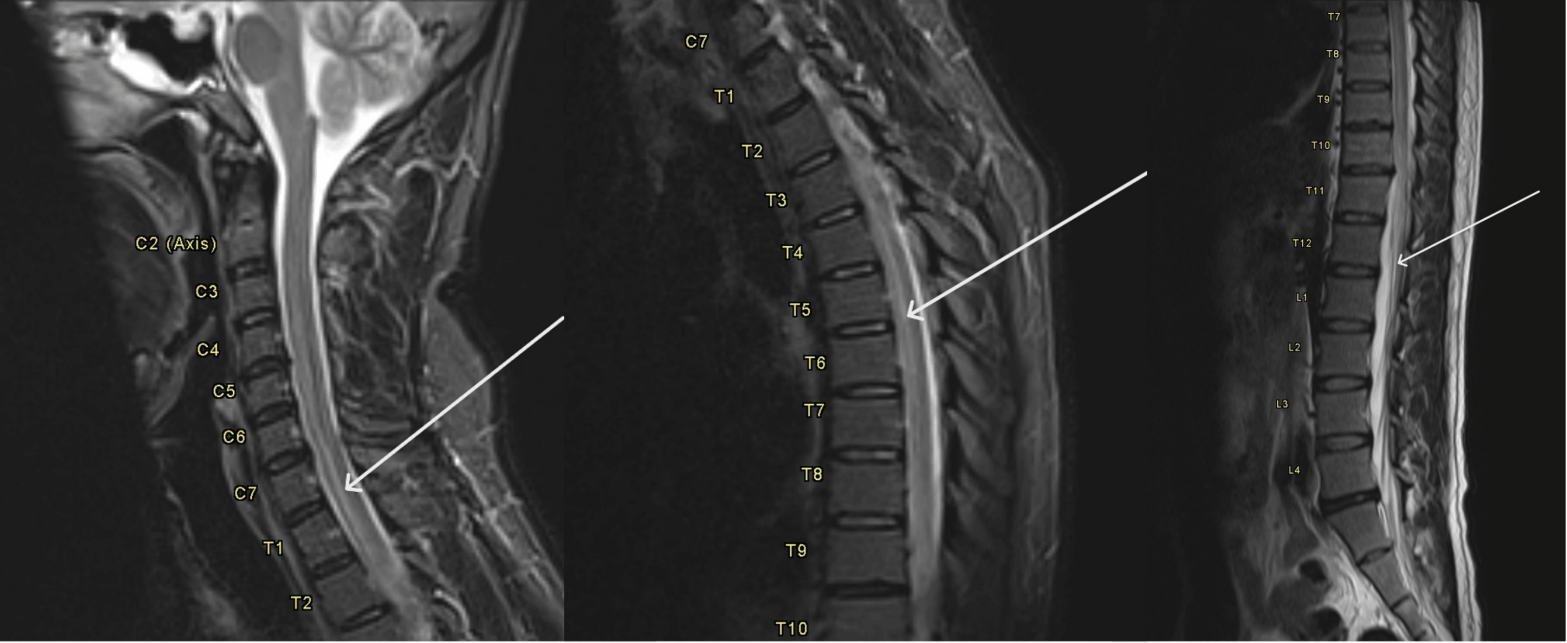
MRI spine showing an inhomogeneous central T2 hyperintense cord signal abnormality extending from C3 to the conus with no associated enhancement, consistent with inflammatory myelopathy.
